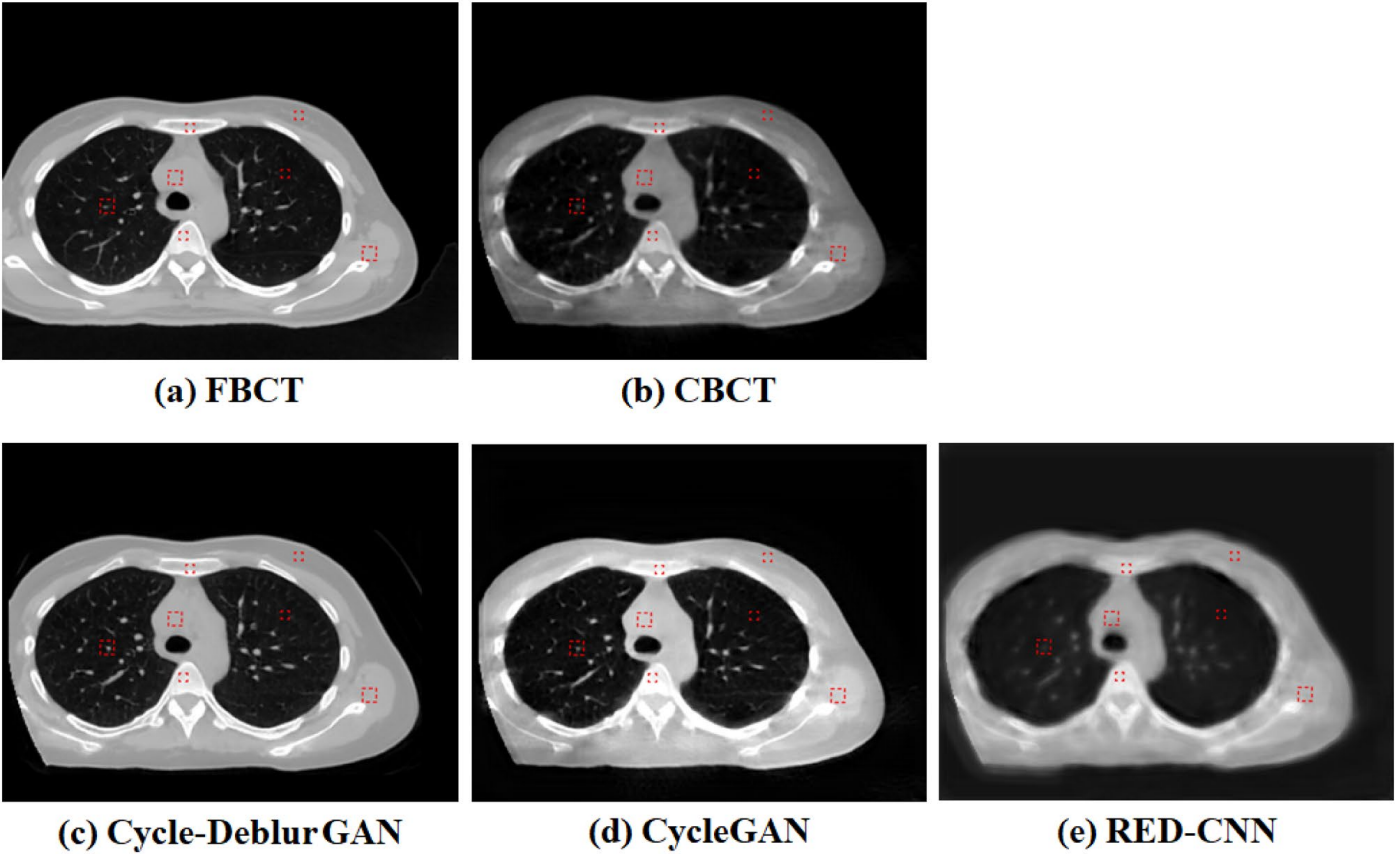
(a) FBCT, (b) CBCT, and the modeling results of (c) Cycle-Deblur GAN, (d) CycleGAN, and (e) RED-CNN were performed by XVI software and PMOD software. (W = 1450, L = − 225 for all CT images).
